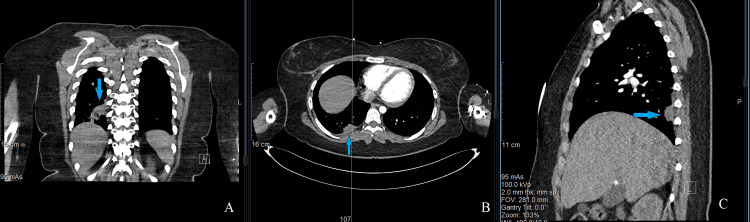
Chest CT after anti-fungal therapy, in three planes Coronal plane (A), axial plane (B), and sagittal plane (C). Arrows showing lung mass of the right lower lobe after antifungal treatment, now measuring 2.9 × 2 × 1.5 cm.
